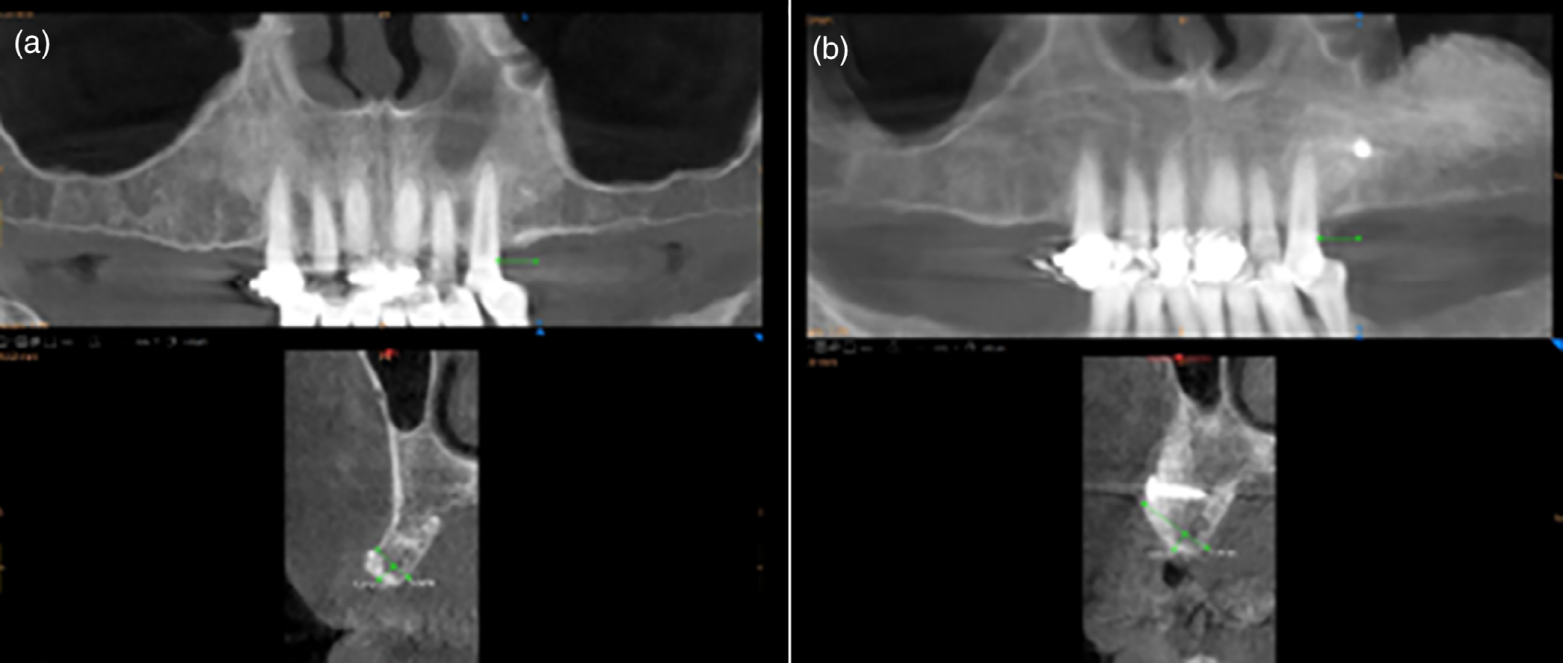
Radiographic analysis with linear measurement of alveolar ridge before (a) and after (b) augmentation
